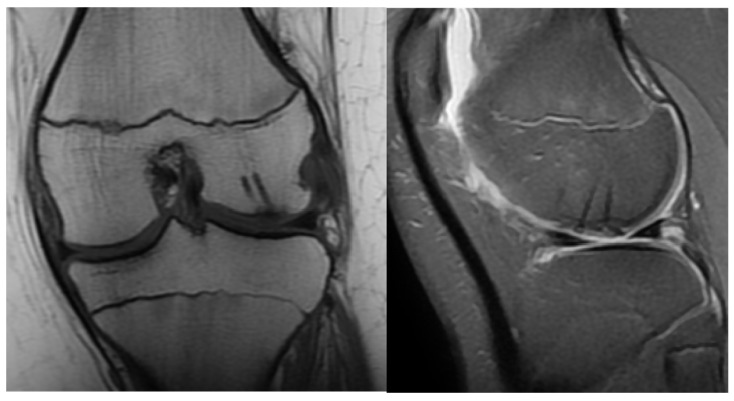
Control MRI one year after surgery. The remainder of the absorbable nails can be seen inside the femoral condyle.
